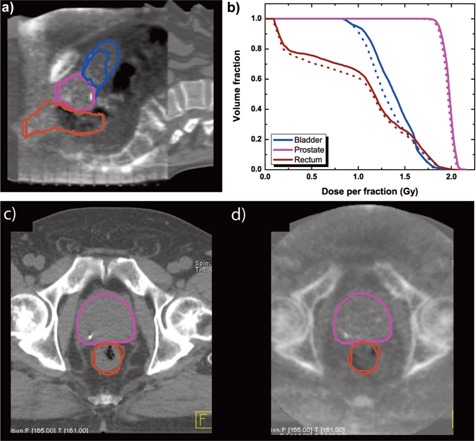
Dose calculation with clinical kVCT and MVCBCT images. (a) Sagittal slice showing the overlaid contours of the prostate (pink), rectum (brown) and bladder (blue) from the planning kVCT and the MVCBCT image. The bigger bladder contour is from the MVCBCT image. (b) Dose‐volume histograms of these structures for treatment planning (dotted lines) and obtained with the MVCBCT image (solid lines). (c) Close up of the prostate and rectum contours on the planning CT image. (d) Close up of the prostate and rectum contours on the MVCBCT image.
